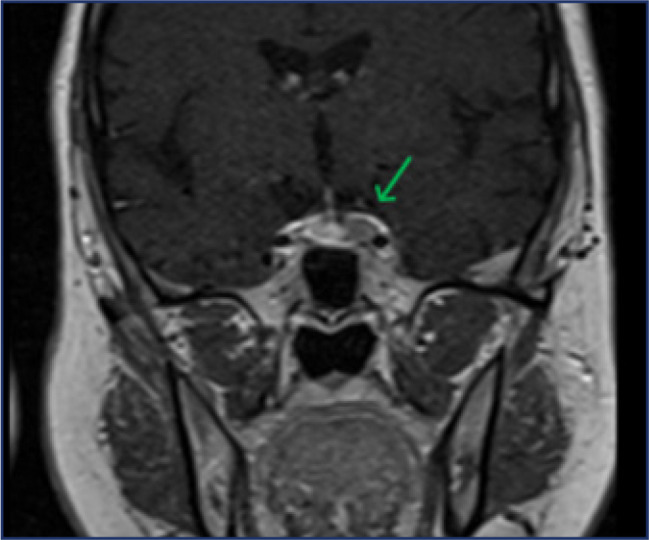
Magnetic resonance imaging of the sella turcica: Coronal T1-weighted
